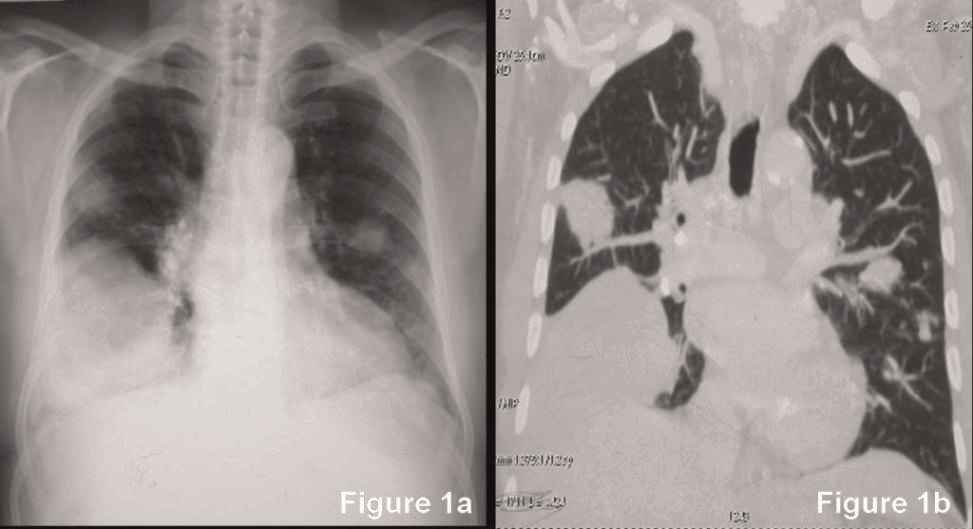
(a) and (b) Chest radiograph and CT scan (coronal section) of chest. Chest radiograph (a) showing a pleural based mass lesion in right lower zone and nodular lesions in right and left mid zones. Coronal section of CT scan of chest (b) showing a large right lower lobe mass and multiple varying size nodules involving left upper lobe, lingula and apical segment of left lower lobe of lung.
